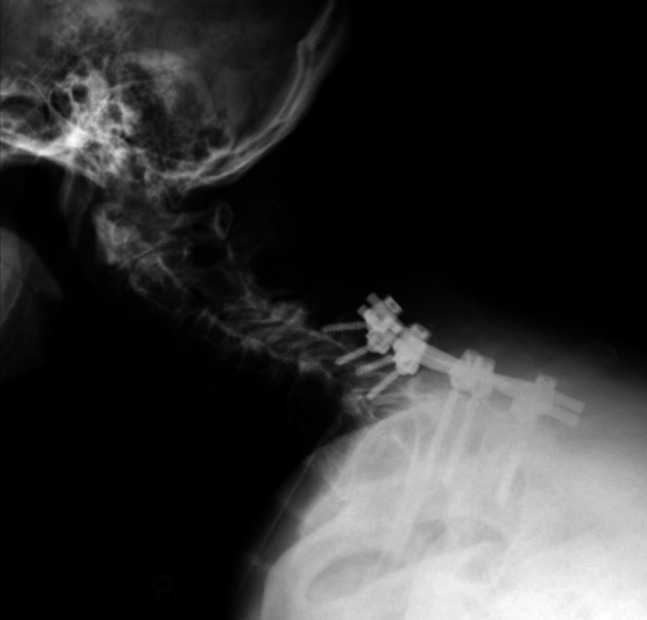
Lateral radiograph of the patient at 6 months after C5 to T2 posterior cervical fixation.
